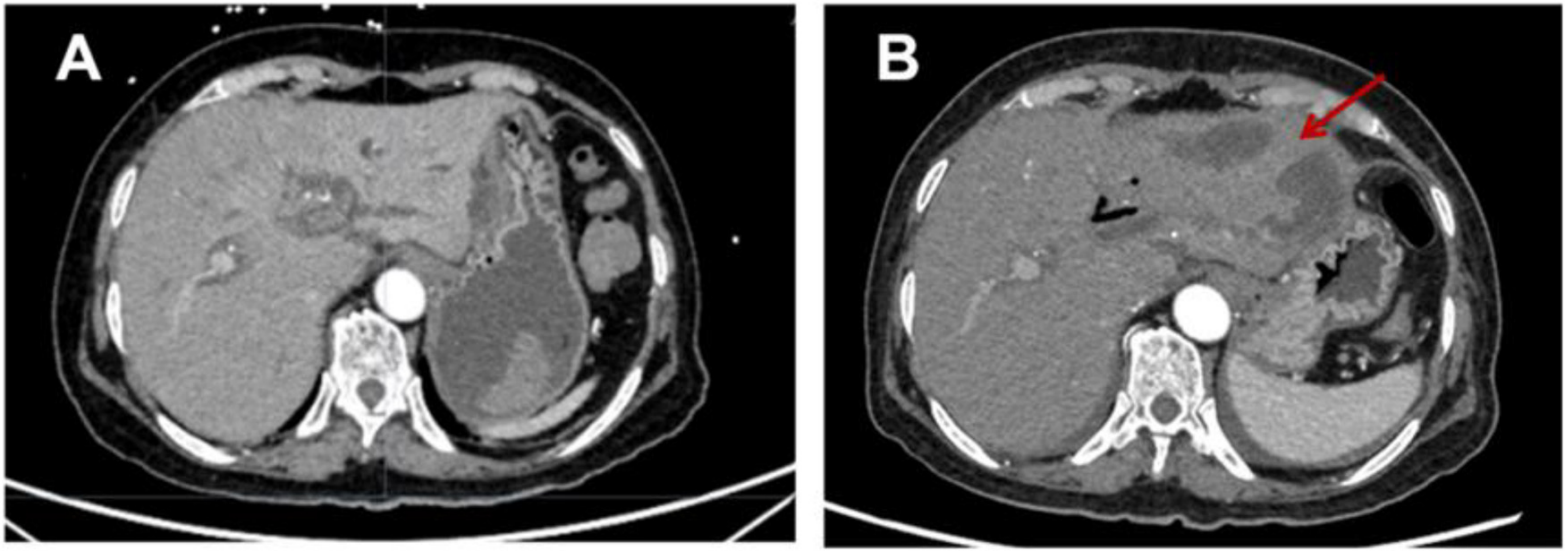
(A) Preoperative hepatic artery embolization and (B) Two weeks after hepatic artery embolization, arterial phase CT showing reduced liver parenchymal density, left hepatic softening, and a liquefied cavity (red arrow).
